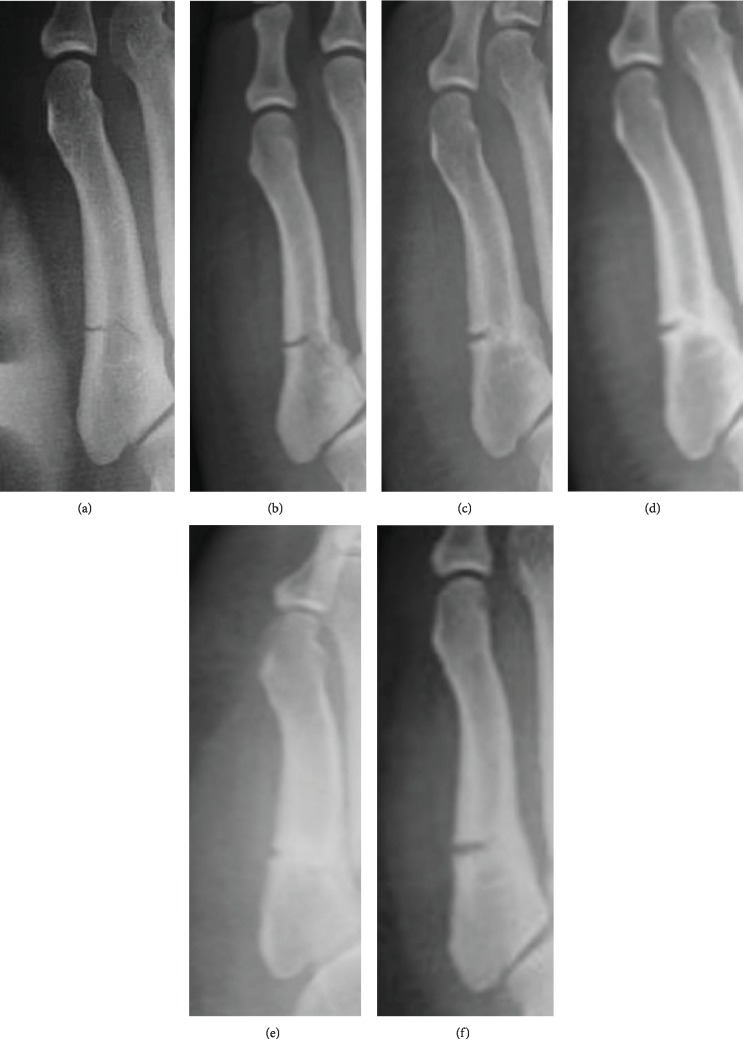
Case 2: anteroposterior radiographs of a 22-year-old sumo wrestler with a Jones fracture (a–f). Radiography shows gradual bone union at 2 weeks (b), 10 weeks (c), 3 months (d), and 4 months (e) after conservative treatment. However, at 6 months after treatment, the foot was refractured (f).
